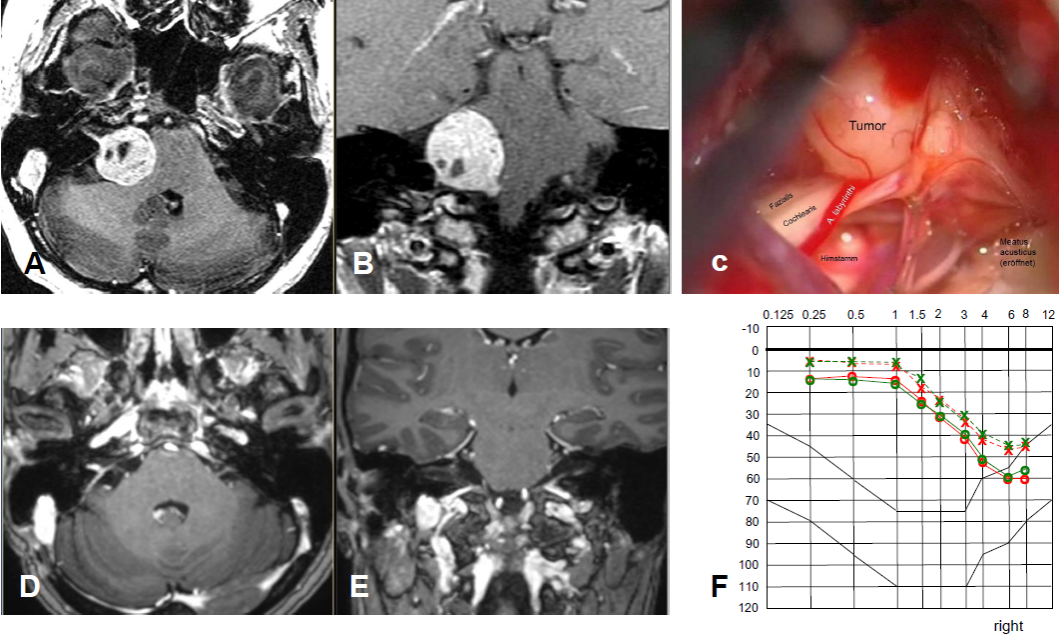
Upper row: preoperative MRI, T1-w + CM; A transversal; B coronal; C surgery site before complete resection of a residual tumor. Facial and cochlear nerves are less adherent, the labyrinthine artery is well seen. Lower row: postoperative MRI, T1-w FS + CM; D transversal; E coronal. Preoperative (green) and postoperative (red) tone audiogram. The linear contrast enhancement at the posterior wall of the right internal auditory meatus and extrameatal meningeal is a consequence of the fistula covering and not a hint to residual tumor.
